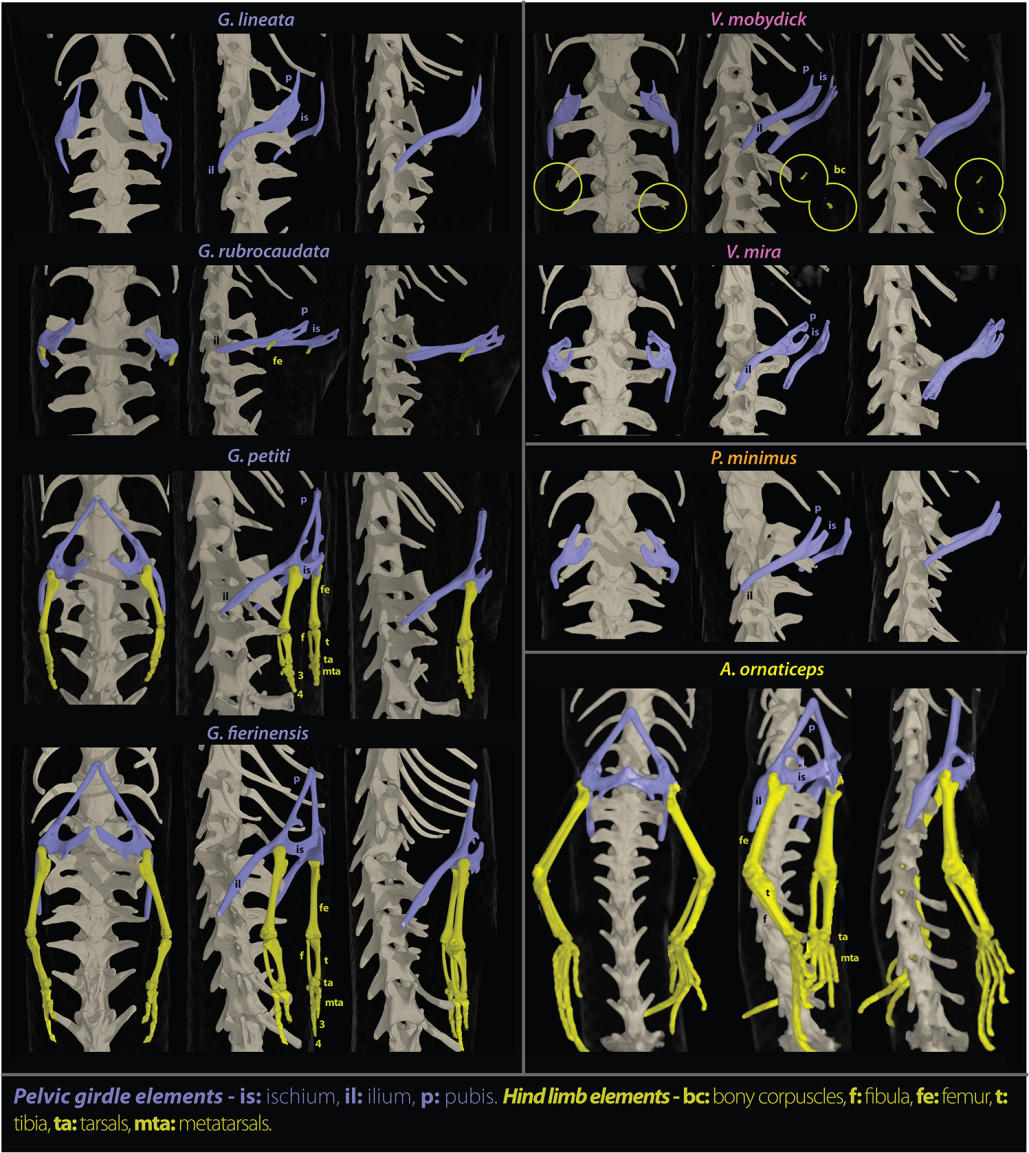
Pelvic girdle and forelimbs skeletal anatomy. Computed tomographic reconstruction of the pelvic girdle (in purple) and hindlimbs (in yellow) of seven different species of blind sand-swimming skinks and one species without limb regression (*Amphiglossus ornaticeps*), based on microtomographic scans, in ventral, ventrolateral and lateral view (not to scale).
